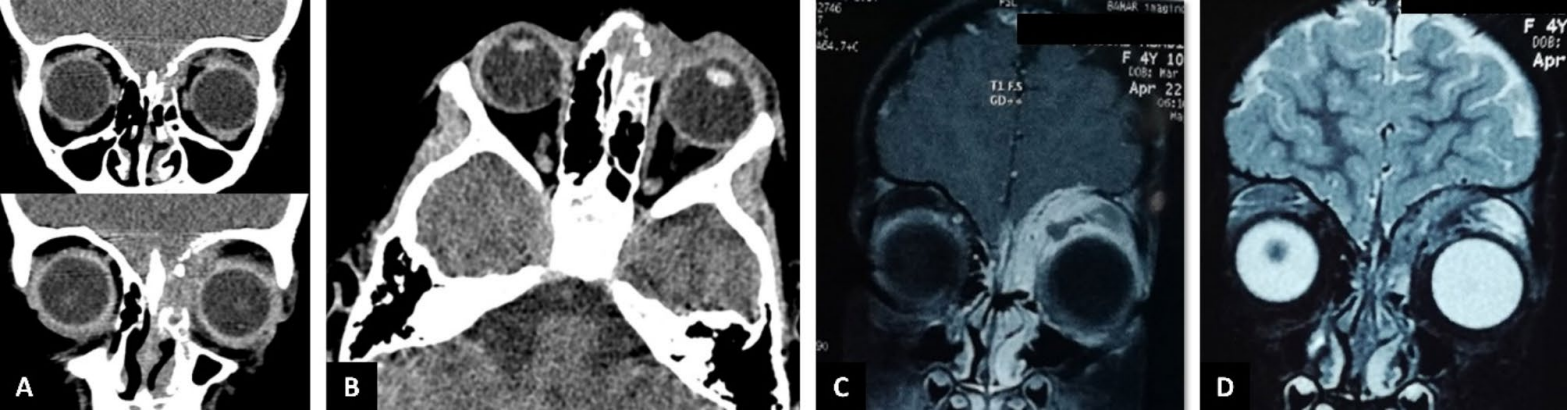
Orbital computed tomography (CT) and magnetic resonance imaging (MRI) scans. A: Coronal CT view showing an infiltrative, extraconal soft tissue mass in the supramedial aspect of the anterior orbit, with bone erosions and destruction in the medial frontal bone extending toward the fronto-maxillary bone suture on the left side. Maxillary sinuses were clear. B: Axial CT view demonstrating fullness of the left anterior ethmoidal sinus. C: Coronal T1-weighted MRI with gadolinium contrast revealing enhancement of the lesion and adjacent dura mater, without obvious intracranial spread. D: Coronal T2-weighted MRI showing the lesion to be iso- to hypointense compared to the extraocular muscles
